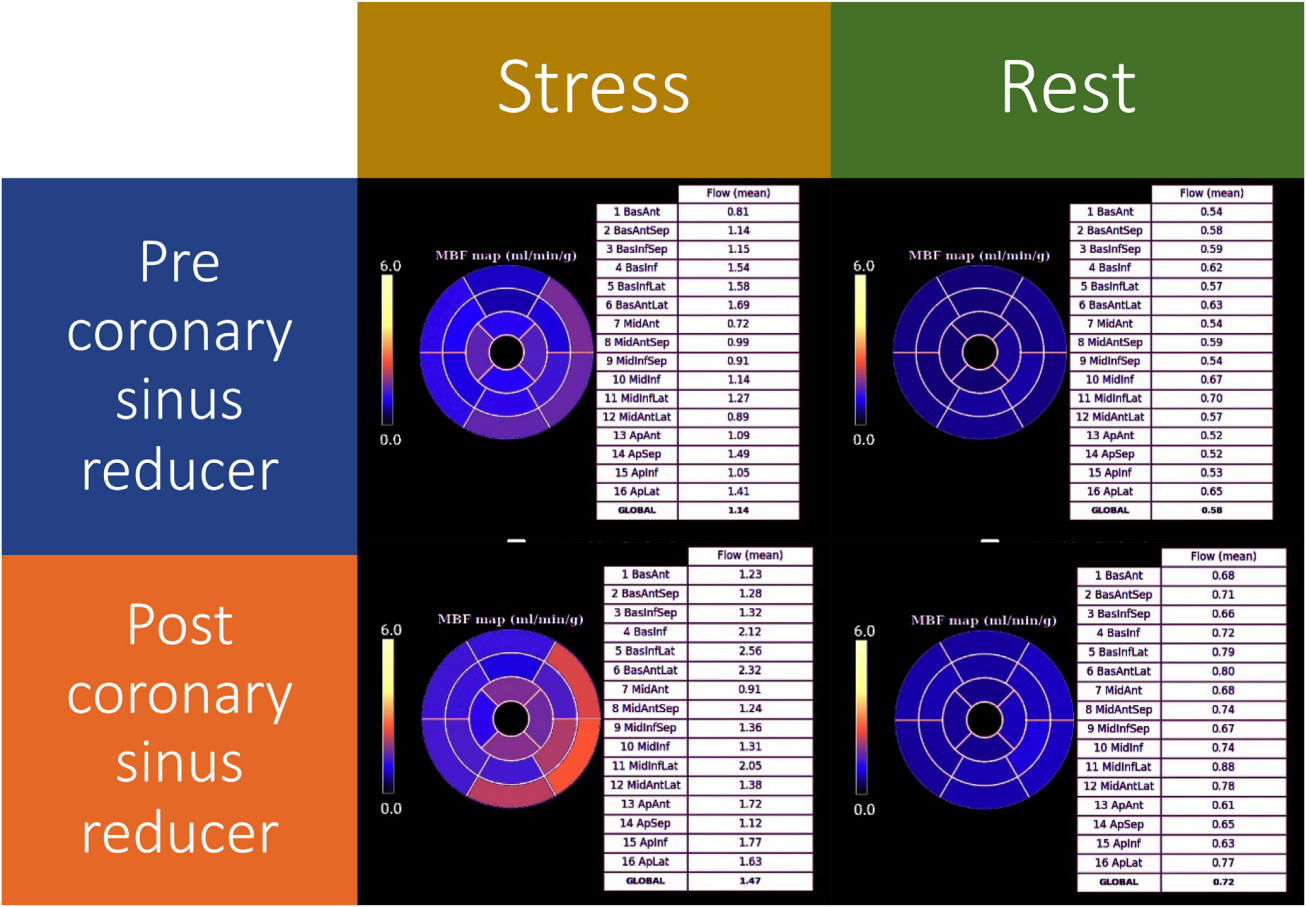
Cardiac magnetic resonance imaging myocardial blood flow maps at stress and rest before and after coronary sinus reducer. On the preprocedural images there is severe global hypoperfusion, most pronounced in the basal anterior wall and mid-septum. After the procedure there is a significant increase in the global blood flow, with the greatest improvements in the regions with the poorest baseline myocardial blood flow.
